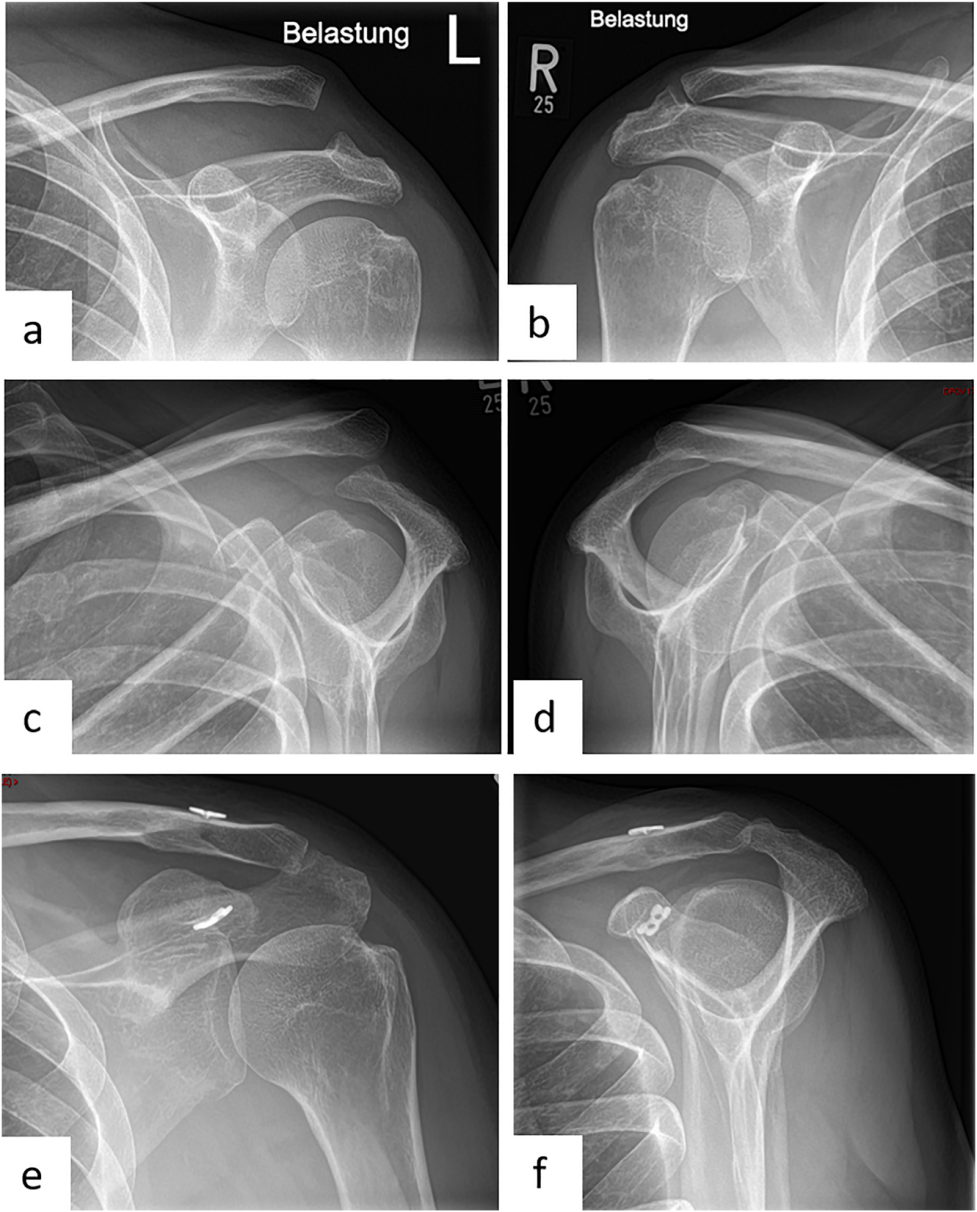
Female patient (50 y), left shoulder, acute acromioclavicular joint dislocation (Rockwood 5) (preoperative X-ray): a – Zanca stress radiographs with 10 kg weights left shoulder, b – Zanca stress radiographs with 10 kg weights right shoulder, c – Alexander radiograph left shoulder, d – Alexander radiograph right shoulder, arthroscopically supported vertical and horizontal stabilization with a TightRope, postoperative X-ray: e – Zanca, f – Outlet view.
